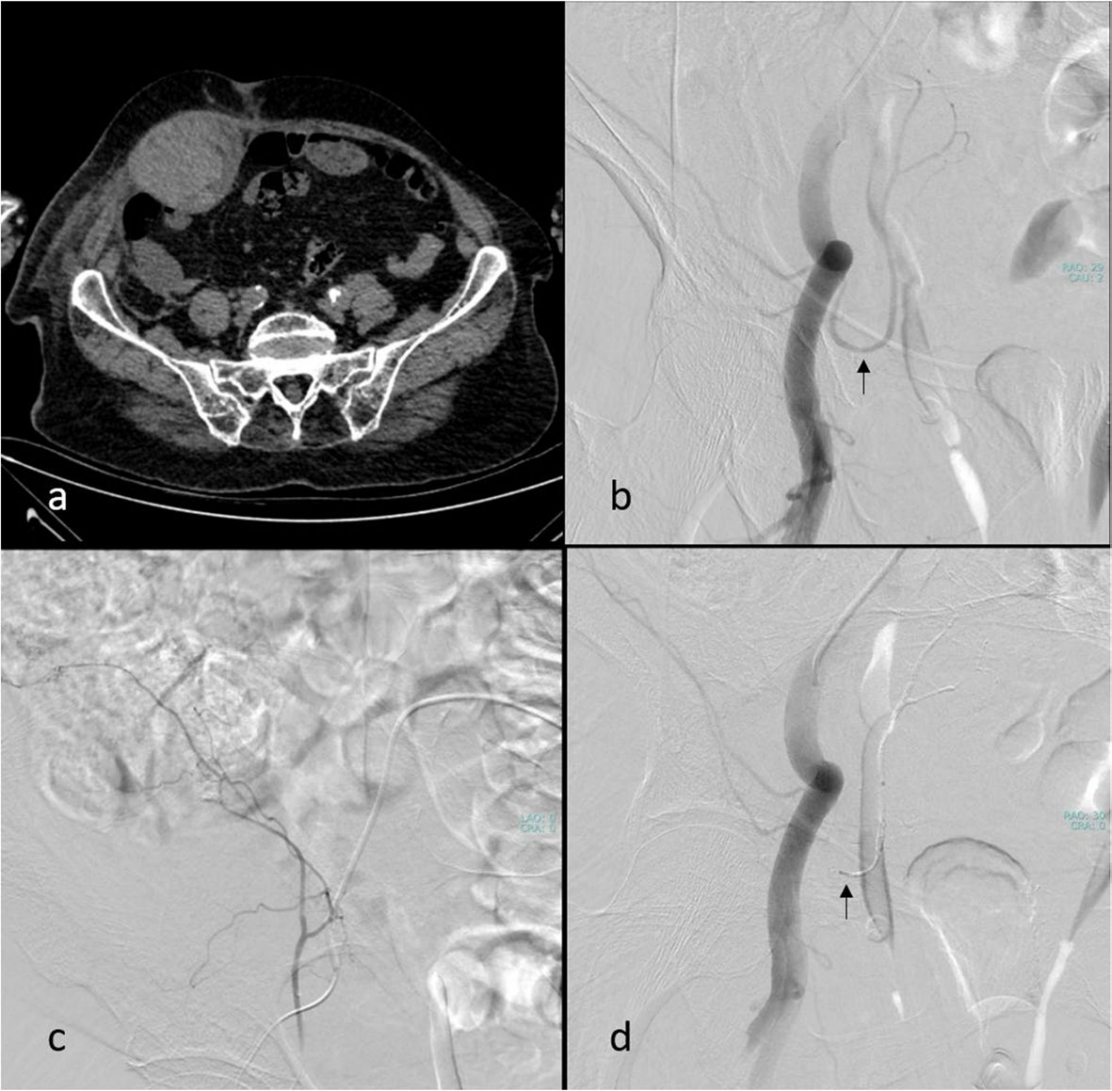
Radiological images of a 77-year-old female patient with a right rectus sheath hematoma. Non-contrast computed tomography image (a). The right external iliac angiogram shows the deep inferior epigastric artery (black arrow), which was accessed via a coaxial microcatheter (b). Despite the absence of active bleeding on imaging (c), embolization was performed using a glue-lipiodol mixture (1:8 concentration) (black arrow) (d)
